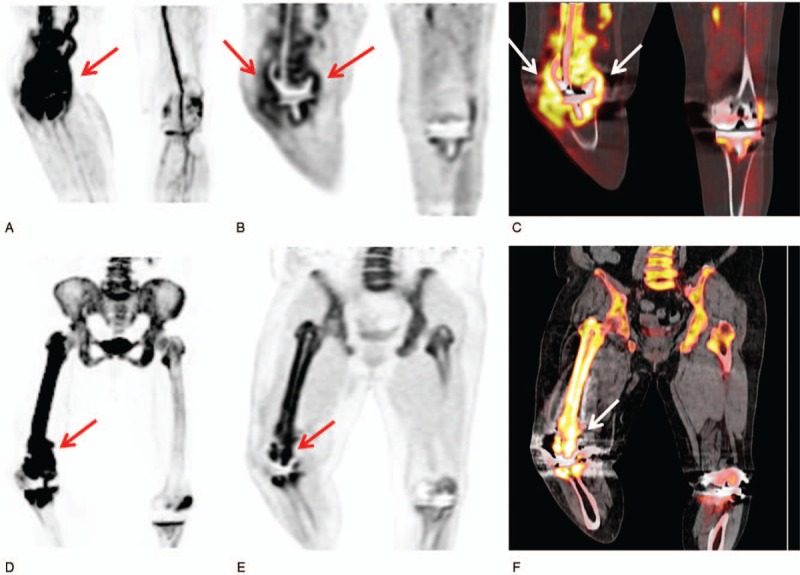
A maximal intensity projection image (A) and coronal PET (B) and fused PET/CT (C) images from the early-phase scan and a maximal intensity projection image (D) and coronal PET (E) and fused PET/CT (F) images from the bone-phase scan in F-18 NaF bone PET/CT of a 68-year-old man. Intensely increased radiotracer uptake around the prosthesis in the right femur and knee joint is shown on both early-phase and bone-phase scan images (arrows in A–F). The patient underwent a revision operation and was diagnosed with surgical site infection of the right femur and knee joint. PET/CT = positron emission tomography/computed tomography.
